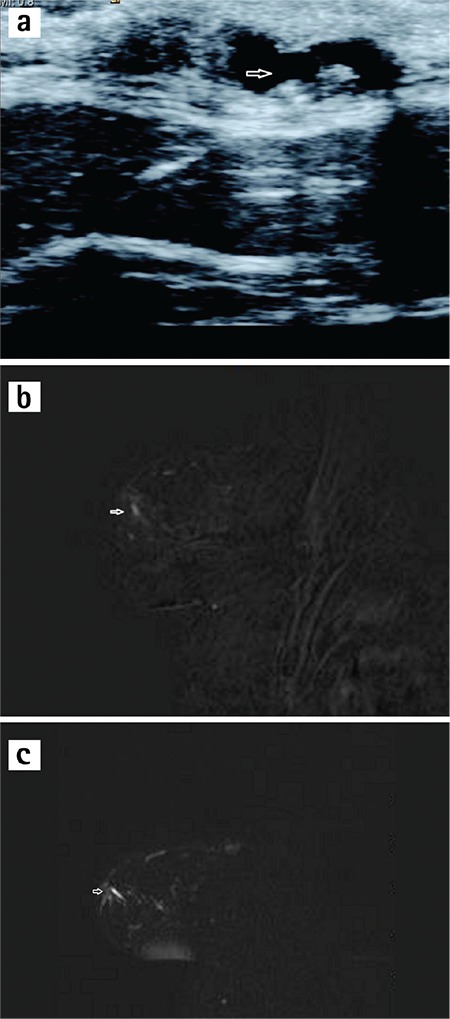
a-c. A woman aged 47 years who presented with bloody nipple discharge. She was diagnosed with papilloma as a result of an operation. Ultrasound image (a) shows multiple well-circumscribed hyperechoic nodules containing millimetric calcific components (arrow) within dilated ducts 5 mm in width. Corresponding axial T1-weighted gadolinium-enhanced subtraction magnetic resonance (MR) image (b) shows non-mass linear contrast-enhancement conforming to trace dilated duct (arrow). In sagittal heavily T2-weighted MR image (c), tubular filling defects in the duct (arrow).
